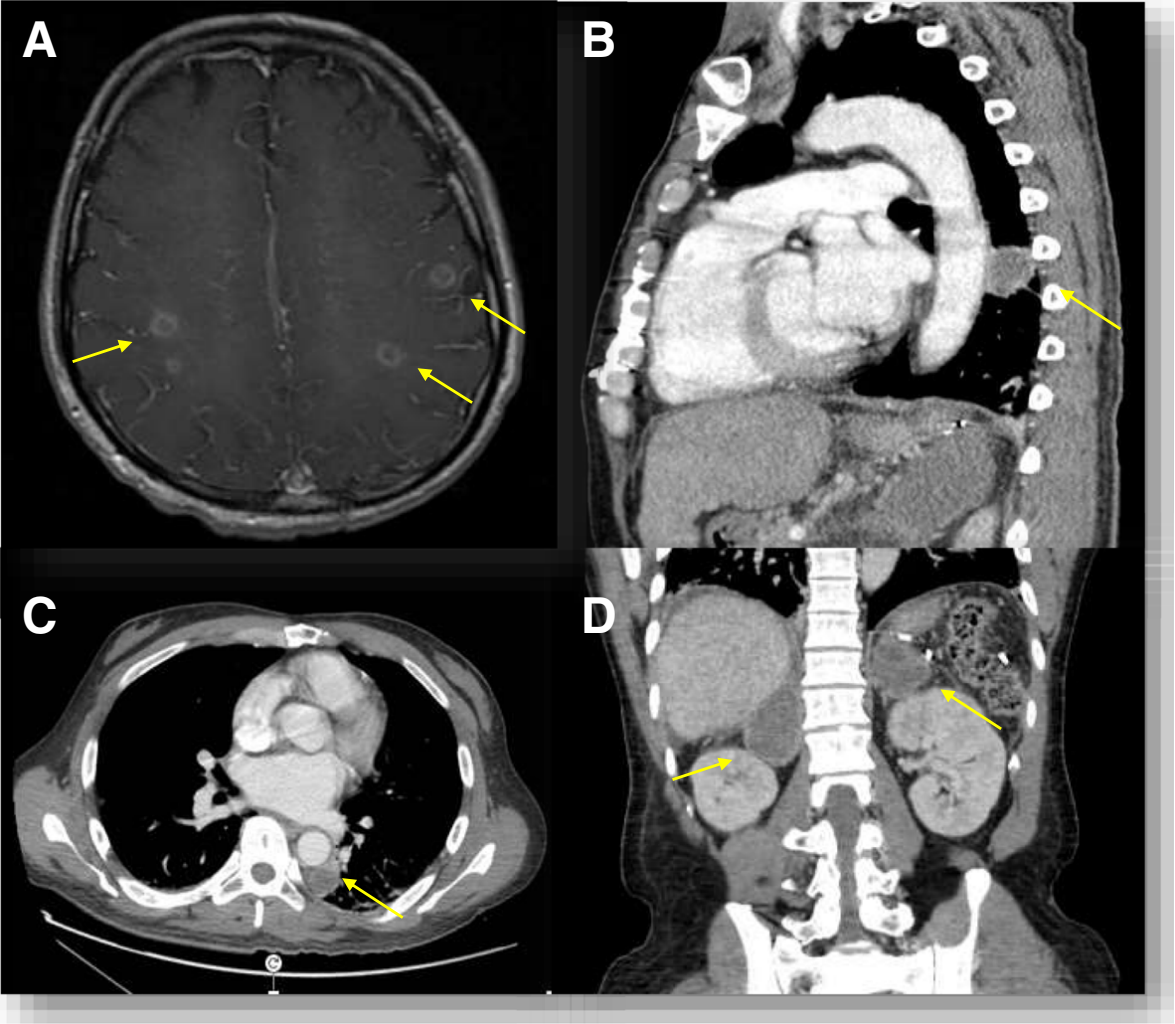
a T-1 weighted brain MRI with gadolinium showing innumerable rim enhancing lesions mostly at the gray-white junction. b and c CT thorax, abdomen and pelvis with contrast demonstrating a retrocardiac rim enhancing mass, measuring 2.7 cm and (d) masses infiltrating adrenal glands bilaterally, left larger than right measuring 8 cm
